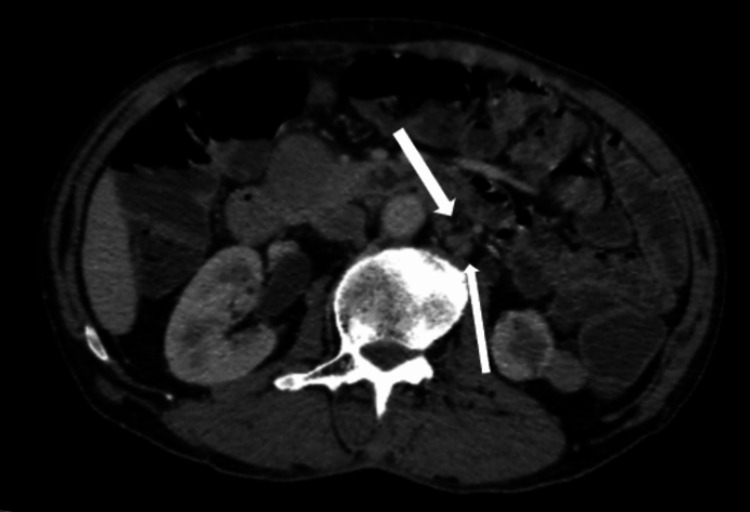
Axial section of contrast-enhanced CT abdomen showing multiple enlarged lymph nodes in the pre and para-aortic region.
